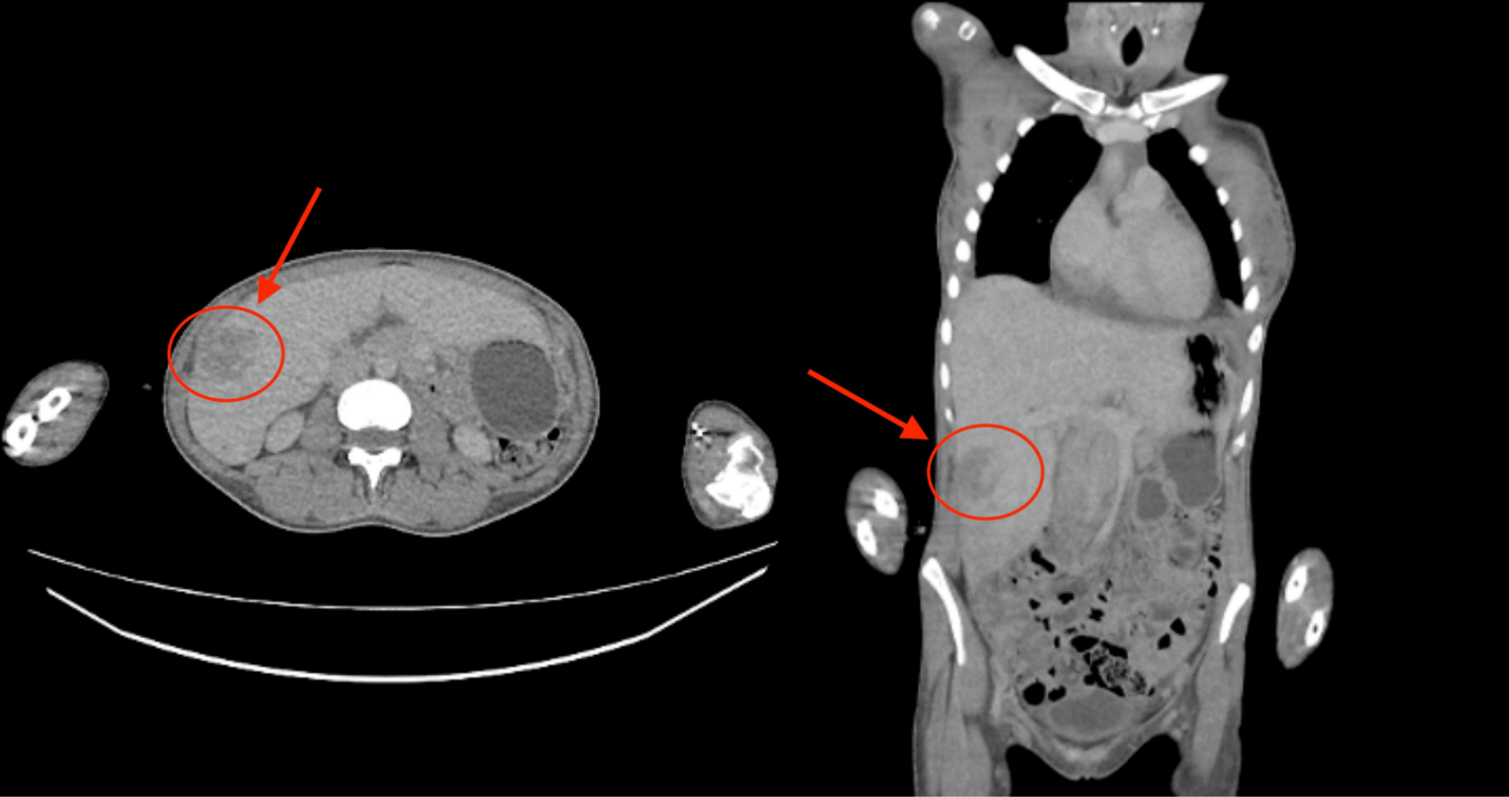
CT images showing a hepatic lesion within the right liver lobe CT: computed tomography
